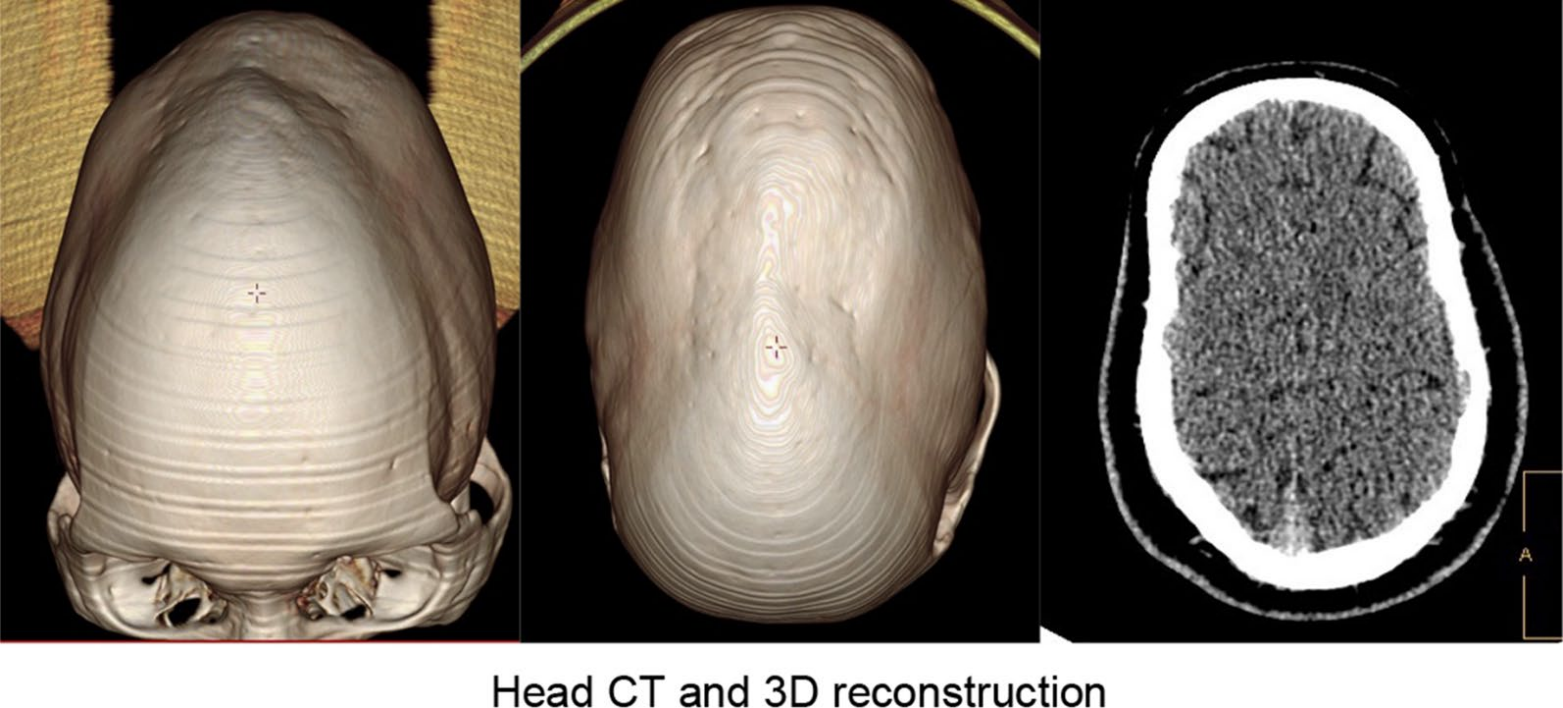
CT scans of the head and a three-dimensional reconstruction of head showing the narrow and elongated shape of the patient’s cranium. The superior region of the forehead appeared pointed and there was a partial upward projection of the skull. The cranial sutures were fused and the overall shape of the head resembled that of a boat
